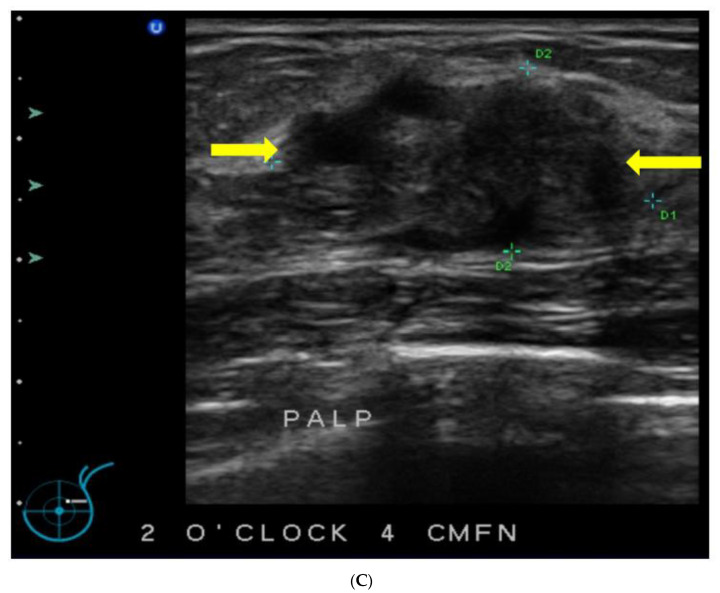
(A) Routine screening mammogram in a 50-year-old woman with screening Density Category D. Calcifications were seen in the left upper inner quadrant (not shown) for which stereotactic core biopsy showed atypical ductal hyperplasia. Surgical excision was planned. Eight months later she returned with a palpable lump in the left upper outer quadrant. (B) Left mammography was performed with a triangular skin marker taped at the site of the lump. The post biopsy marker clip was seen (arrow), but no abnormality was seen at the site of the lump on full field digital mammography (FFDM) or on digital breast tomosynthesis (not shown). (C) Ultrasound showed a 3.2 cm mass (arrows) and abnormal axillary nodes (not shown). Core biopsy confirmed invasive ductal carcinoma. This is an interval cancer.
